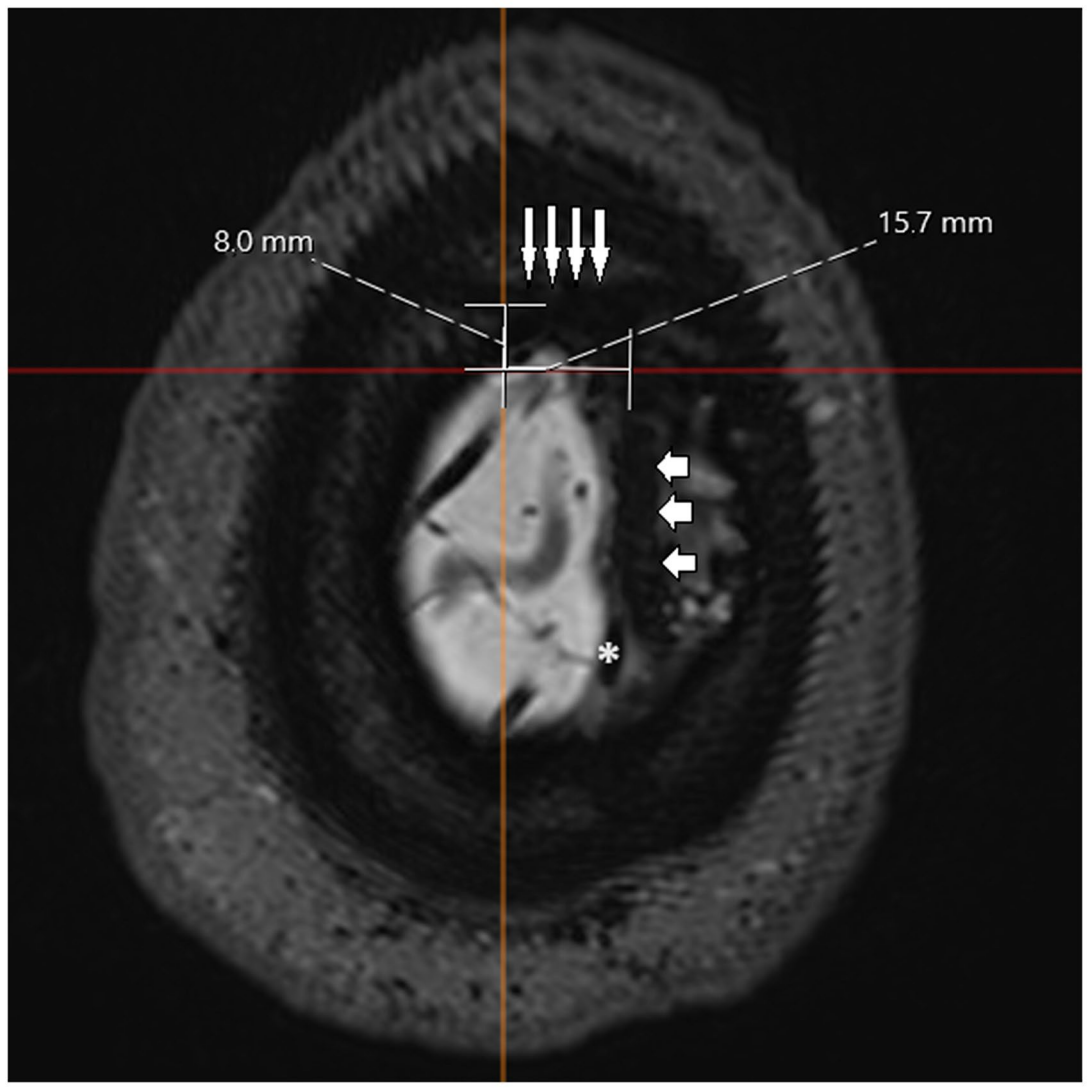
T2 SPACE MRI imaging showing a transversal view of the brain hemispheres and part of the skull of a non-hydrocephalic patient with Parkinson’s disease. The sagittal suture is marked with short, horizontal arrows, and the coronal suture is marked with long, vertical arrows. Due to the nature of the plane used, it is impossible to show the entire skull with both sutures at the same time, and part of the brain hemispheres are visible. The sagittal suture can be seen overlapping the superior sagittal sinus (marked as *). The longest horizontal and vertical lines were drawn to intersect at the established entry point. From this intersection, the perpendicular distance was measured relative to the coronal suture (vertical white line) and the sagittal suture (horizontal white line). In this case, the measured distances were 8.0 and 15.7 mm, respectively.
